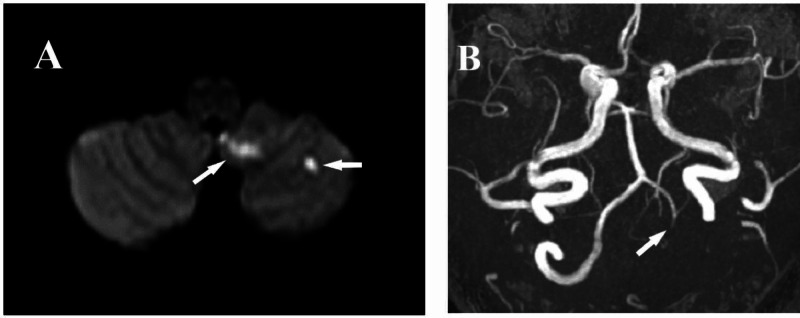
MRI and MRA findings – case 1 (A) Brain MRI, DWI sequence showing multiple restricted diffusion lesions in the left cerebellar region (arrows). (B) Brain MRA showing that only the V4 segment is visualized, while V1-V3 segments are absent (arrow) MRI: magnetic resonance imaging; DWI: diffusion-weighted imaging; MRA: magnetic resonance angiography
